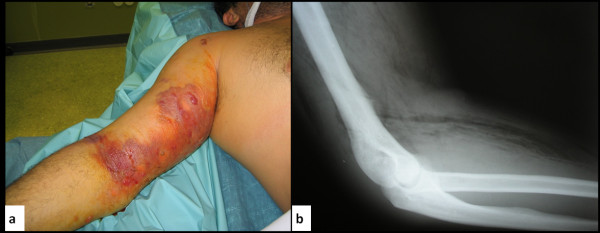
Gas gangrene in an illicit drug user.  a. One and half hours after his admission in the emergency department. b. X-ray of the affected limb revealing gas in soft tissues.
